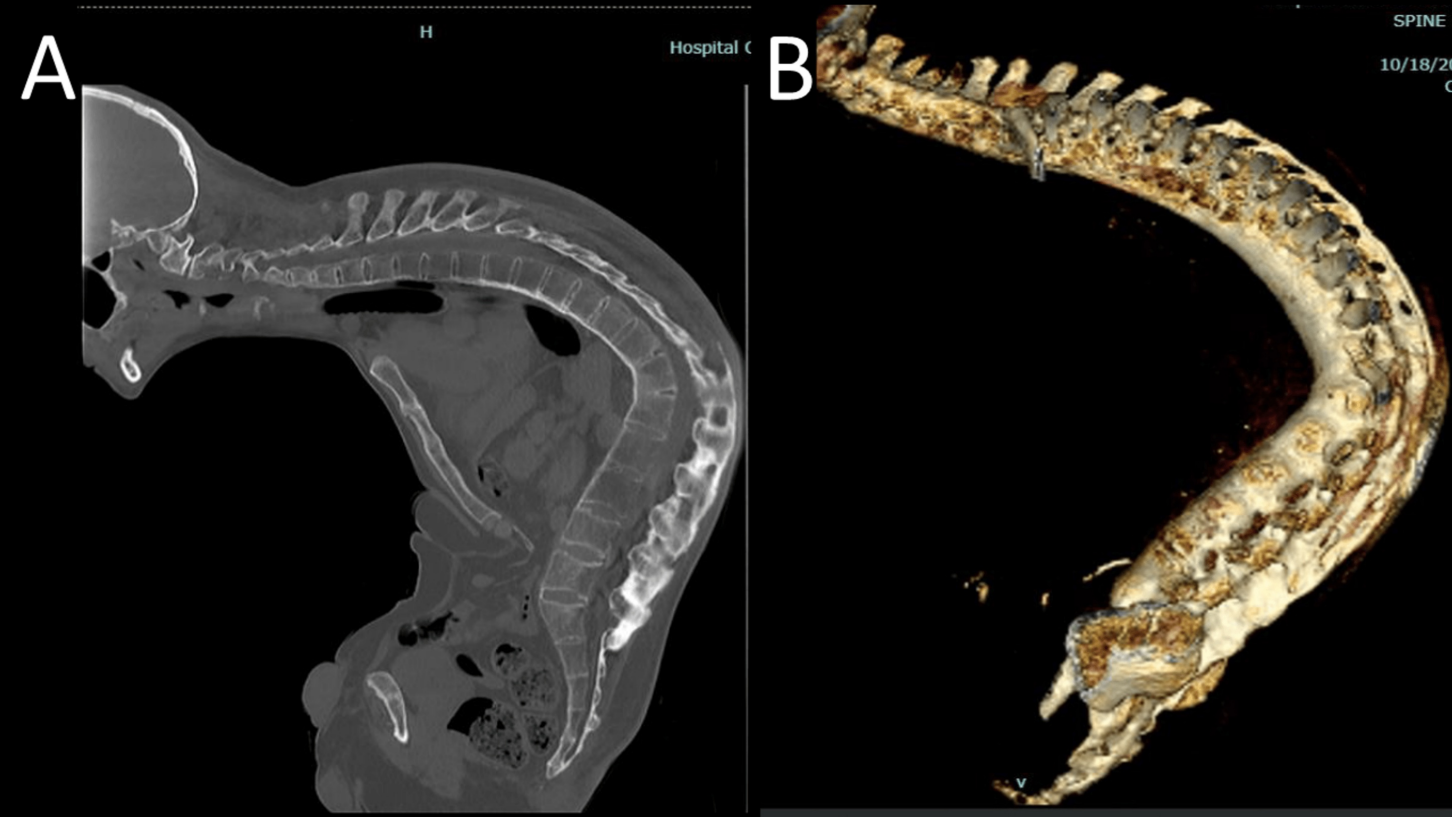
Spinal CT obtained before operation Figure 3A: A CT scan (sagittal view) of the patient showing an ankylosed spine; Figure 3B: Three-dimensional reconstructions of CT scan of the ankylosed spine of the patient
